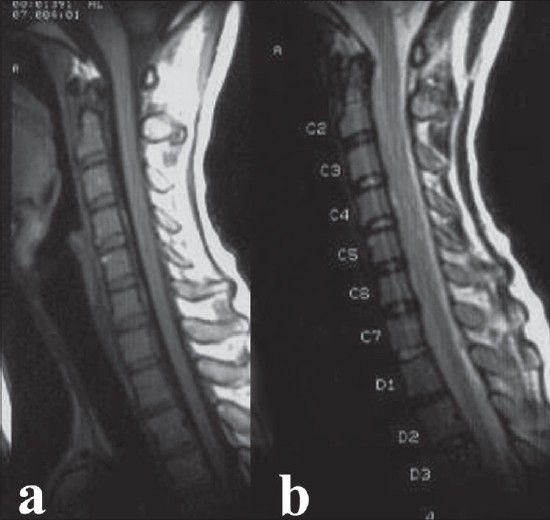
Mid sagittal T1 (a) & T2 (b) weighted MRI of cervical spine 11 days after first MRI shows complete resolution of hematoma
